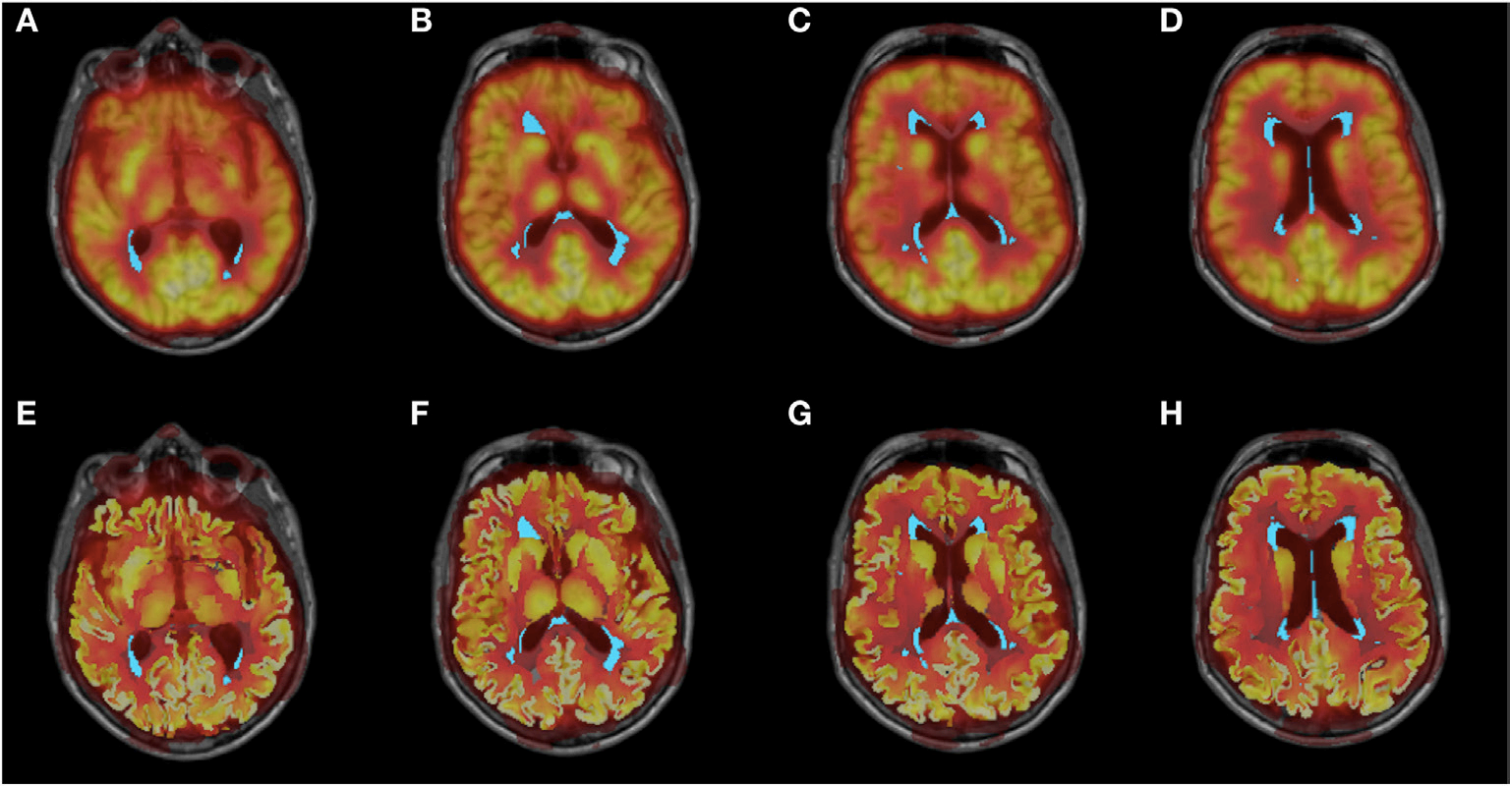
Co-registered structural MRI, WMH segmentation (cyan), and partial voluming uncorrected (A–D) and corrected (E–H) FDG-PET images.
